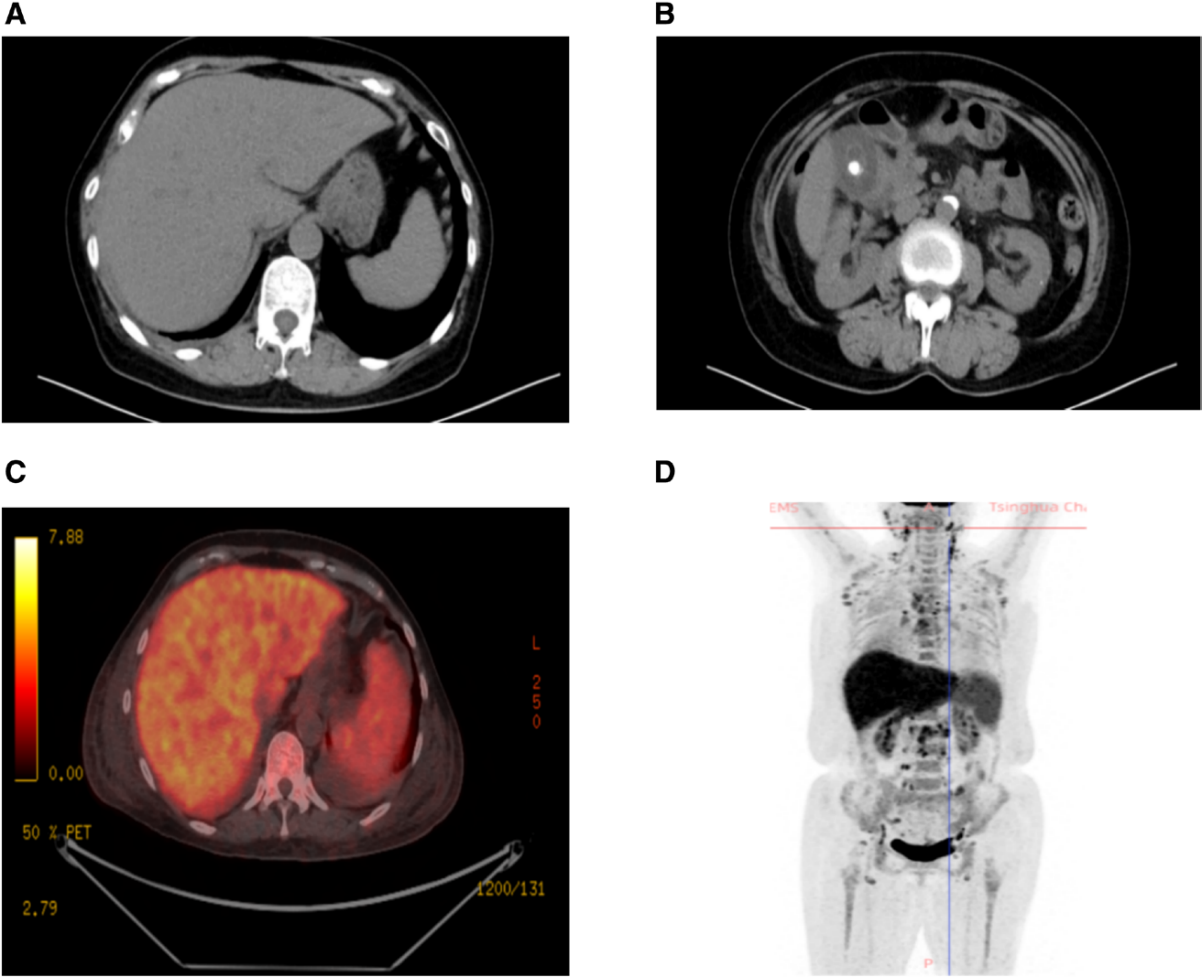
(A) No obvious liver space occupying lesions are seen in this computed tomography. (B) Gallbladder stones are visible in this image. (C) Significantly increased hepatic radioactivity uptake in this positron emission tomography. (D) Multiple lymph node enlargement accompanied by increased radioactivity uptake throughout the body in this positron emission tomography.
